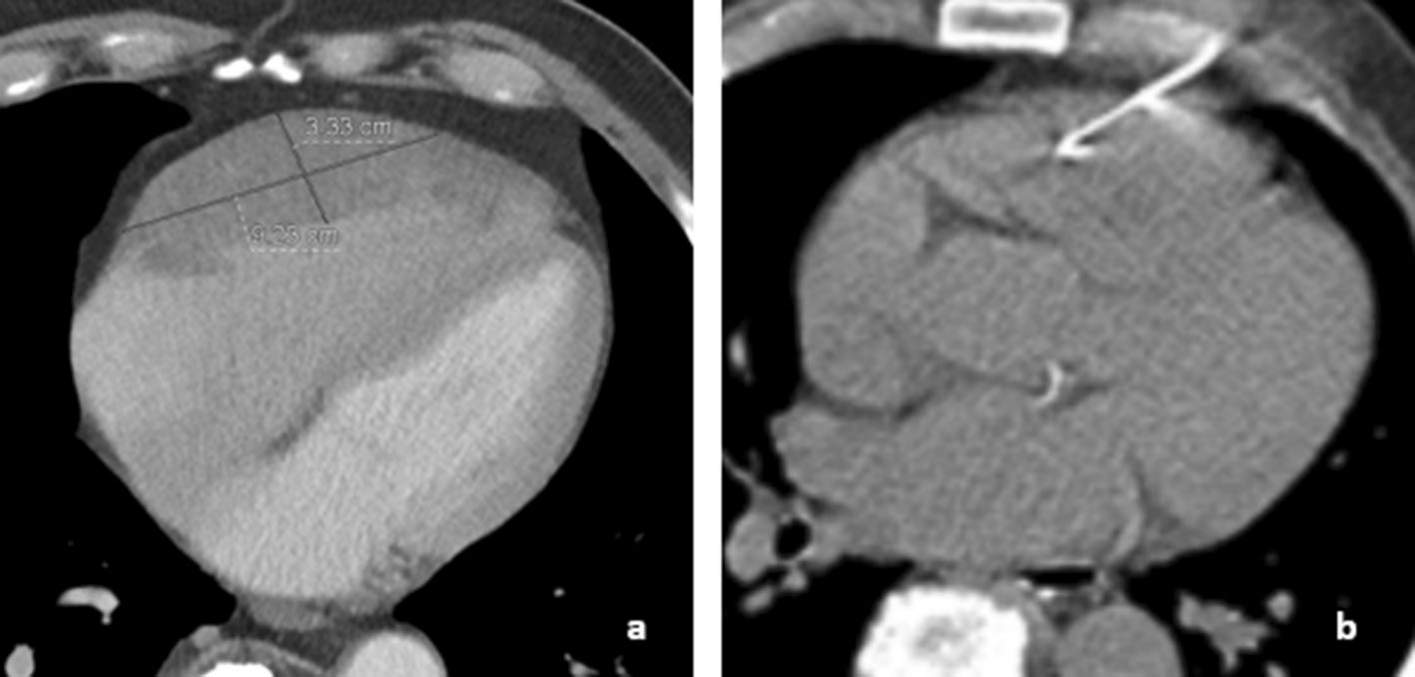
a CT Thorax shows a large mass (9.2 × 3.3 cm) along the anterolateral wall of the right ventricle. b CT-guided puncture of the free wall of the right ventricle with a 18G-puncture needle
